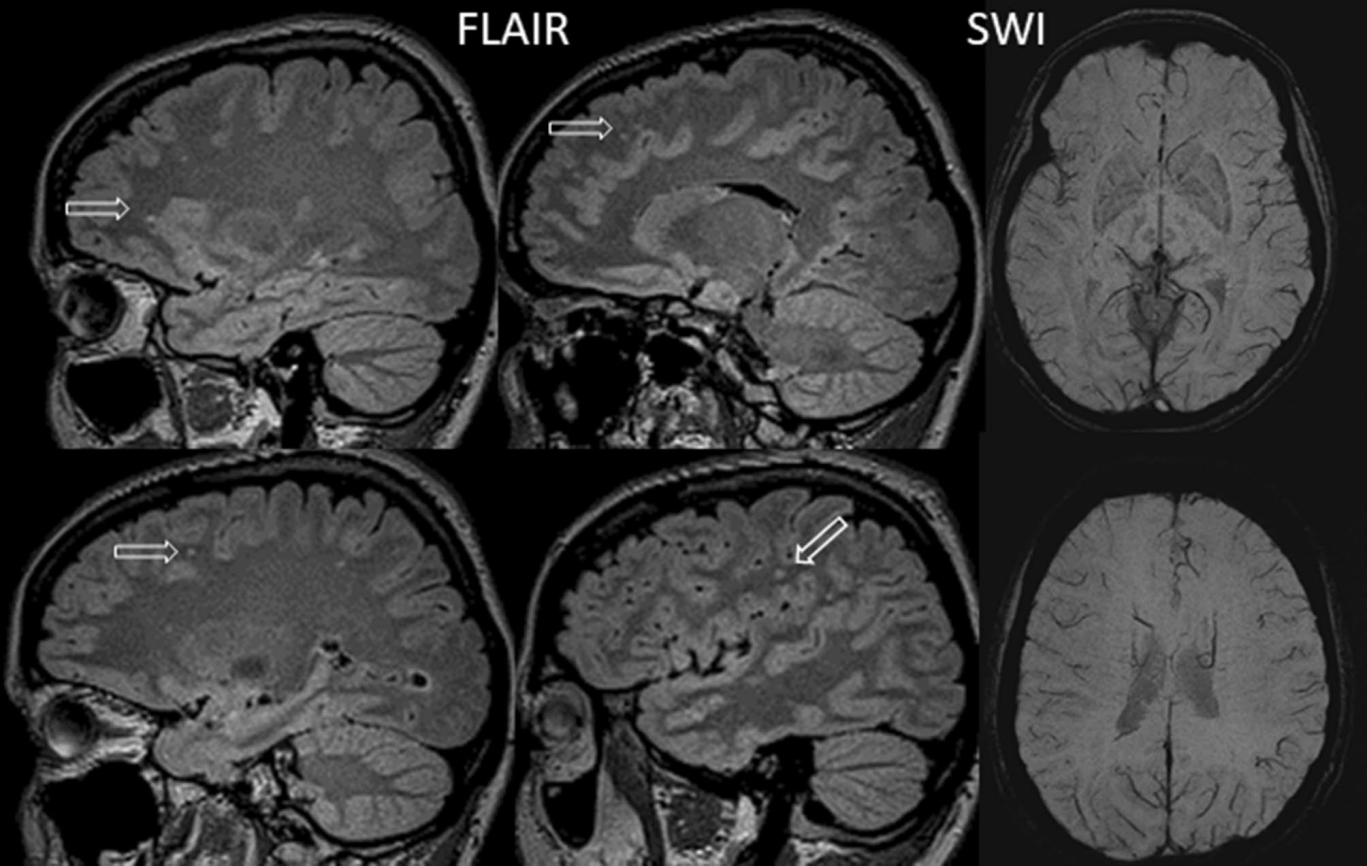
33-year-old female patient, presented with migraine with aura for 10-year duration. Sagittal FLAIR MRI image shows punctate hyperintensities in the white matter within the frontal and parietal lobes (1–2 mm). There are no microbleeds on SWI images.
